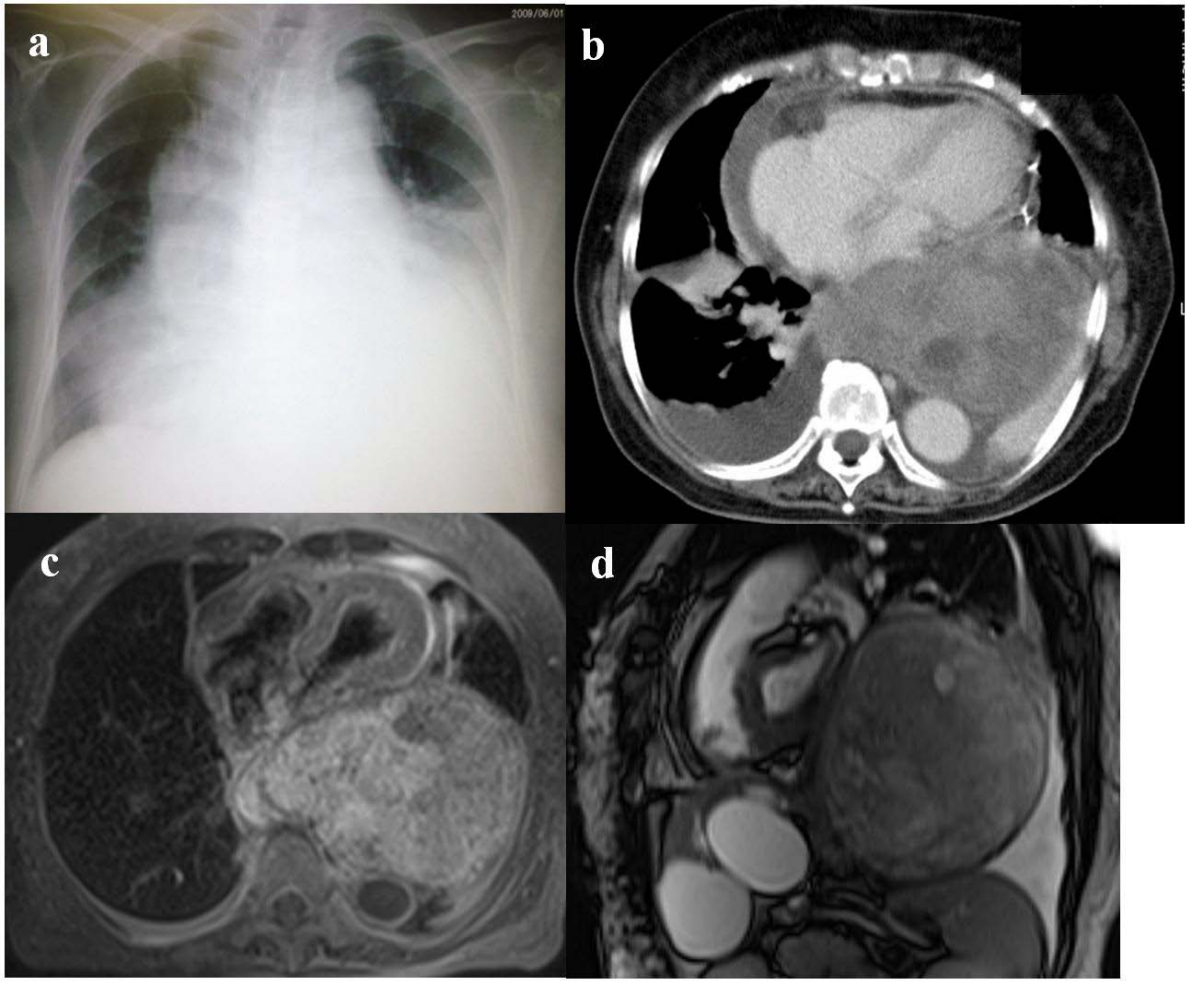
Lung imaging. (a) Chest radiograph obtained on the day of hospital admission showing bilateral pleural effusion, mediastinal widening and cardiac enlargement. (b) Contrast-enhanced chest computed tomography image (lung window) taken during pericardial drainage, showing a giant posterior mediastinal tumor, pericardial effusion and bilateral pleural effusion. (c) Transversal T1-weighted magnetic resonance imaging (MRI) scan of the chest taken after pericardial drainage, showing a giant encapsulating tumor in the posterior mediastinum compressing the heart. (d) Sagittal True SSFP (steady state free precession) MRI image, showing the tumor occupies most of left thoracic cavity.
